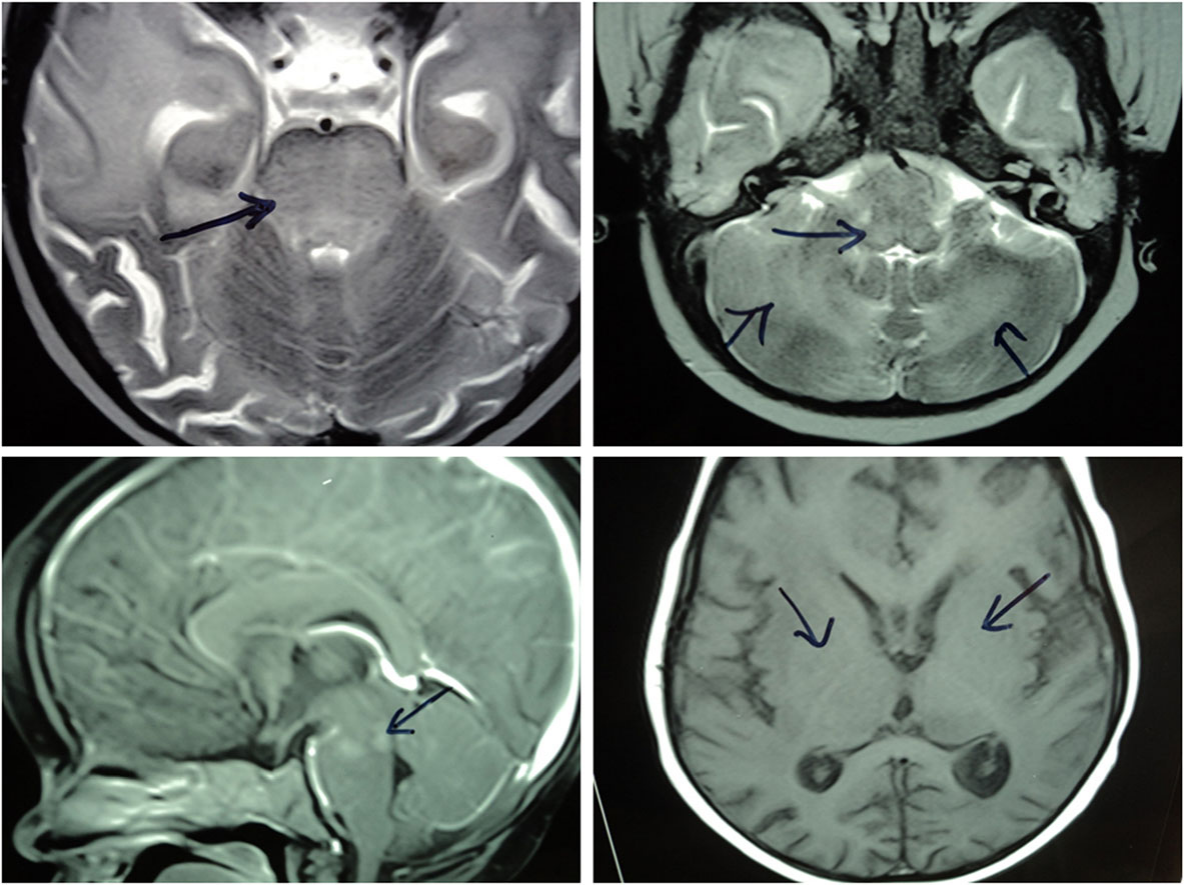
MRI Image of proband (P2). Meningoencephalitis with post-infective extensive demyelination is seen
